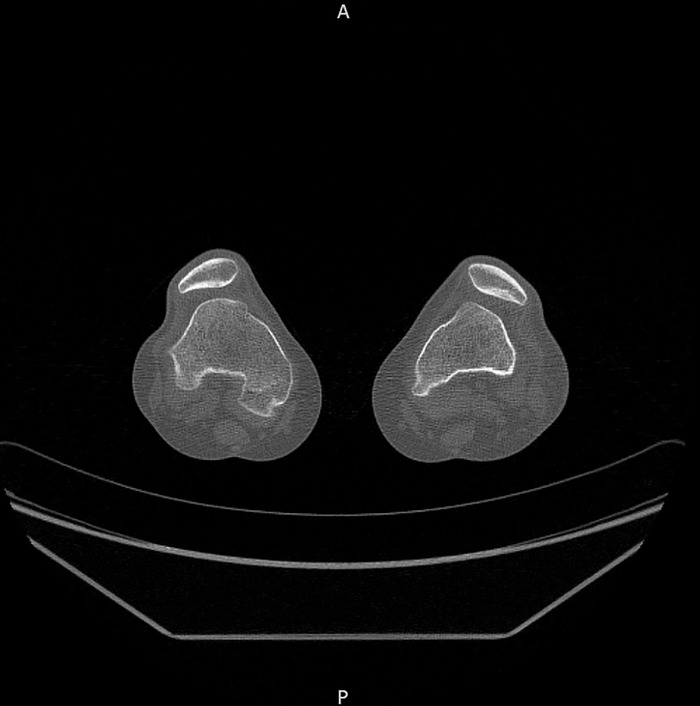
Preoperative axial computed tomography scan of the knees shows bilateral excessive femoral anteversion measuring 25° on the right and 24° on the left. The tibial tubercle–trochlear groove distances are 21 mm on the right and 25 mm on the left.
